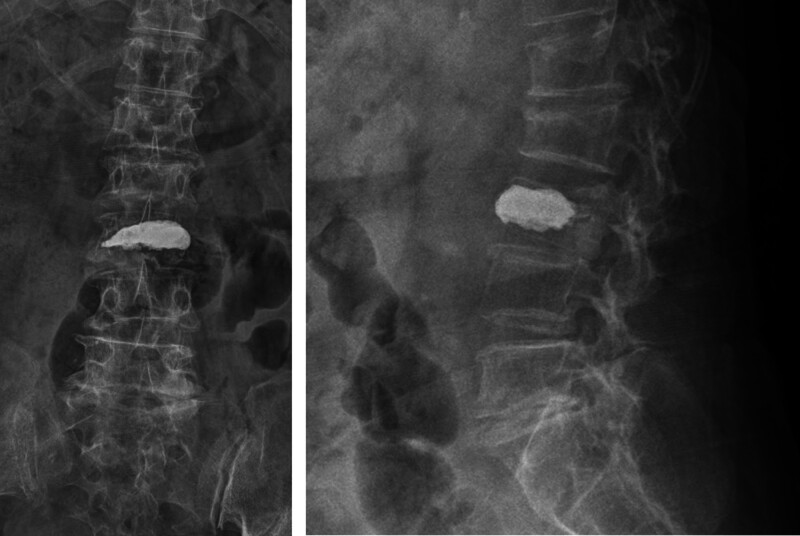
Post-vertebroplasty anteroposterior (AP) and lateral radiographs of the lumbar spine. (A) AP and (B) lateral views demonstrate adequate cement filling within the L3 vertebral body, with bilateral extension covering the posterior two-thirds of the vertebral structure.
